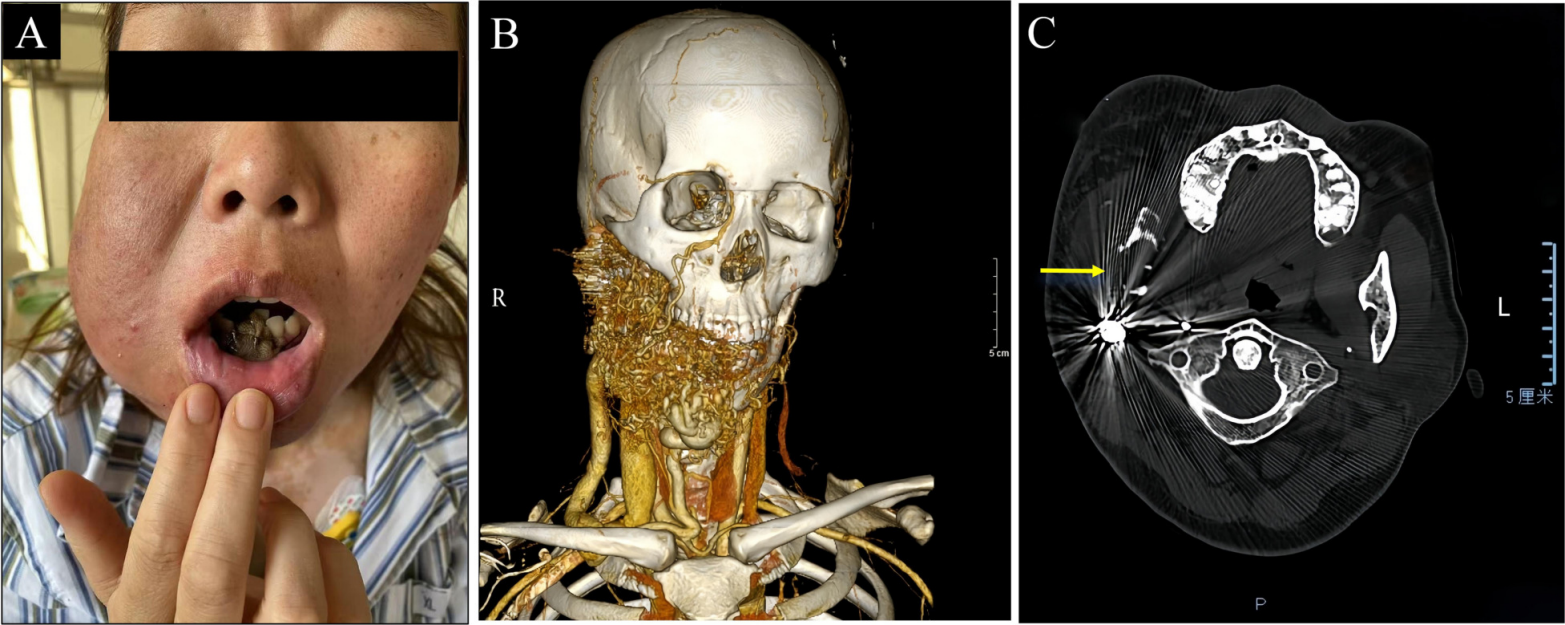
Preoperative characteristics of the patient. (A) Preoperative facial appearance of the patient. (B) Three-dimensional reconstruction of head and neck computed tomography angiography. (C) Head computed tomography scans.
